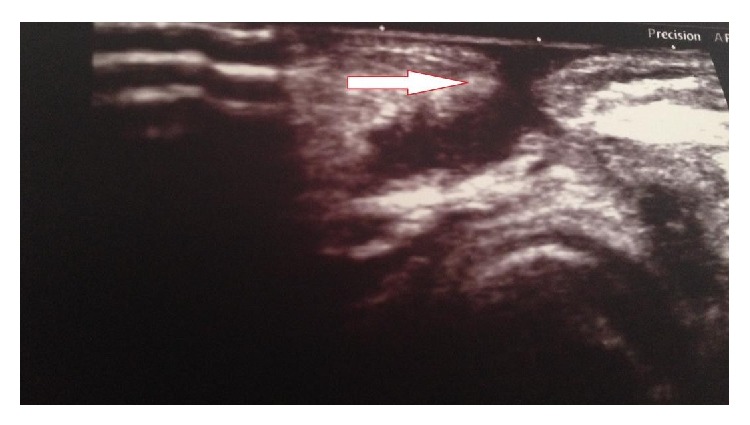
Ultrasonographic image of the subcutaneous sinus tract.
